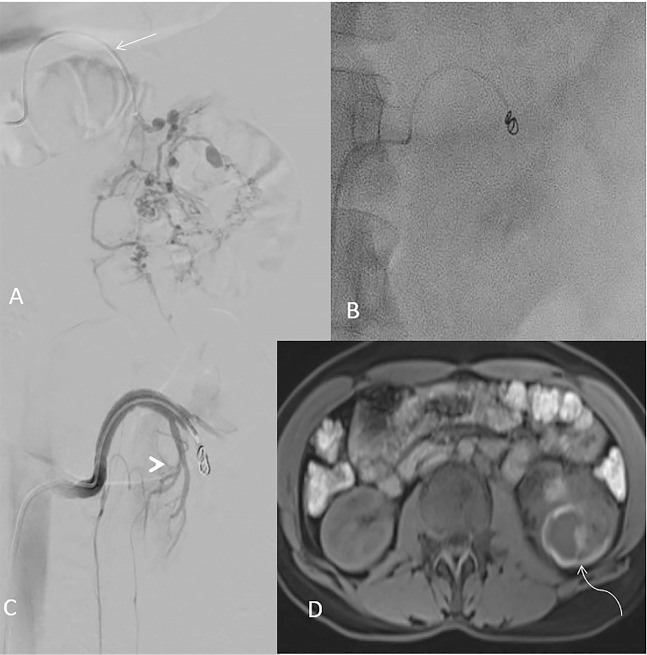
A) Digital subtraction angiography run from left accessory renal artery (straight arrow) showing large angiomyolipoma with multiple intrasac pseudoaneurysms embolized using PVA particles. B) Fluoroscopic image showing coiling of feeder artery. C) Post-coiling angiography showing superselective and complete embolization of angiomyolipoma. Supply to normal renal parenchyma preserved (arrow head). D) Post-embolization (at 3 months) MRI T1W imaging showing reduction in tumor size (curved arrow).
